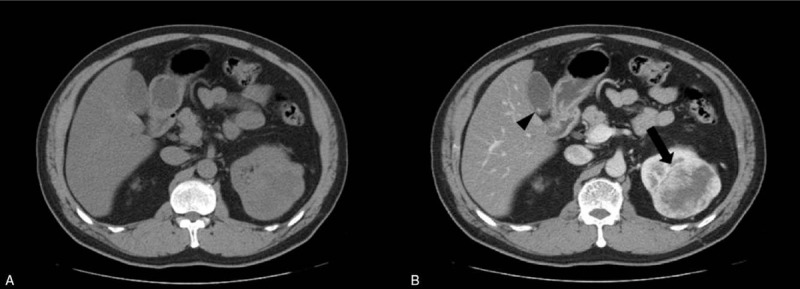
Computed tomography (CT) performed just before nephrectomy. (A) There are no perceptible gallbladder lesions in this unenhanced CT image. (B) Porta-phase axial CT shows a 9-cm tumor at the upper pole of the left kidney (arrow) and 5-mm enhancing gallbladder polyp (arrowhead).
